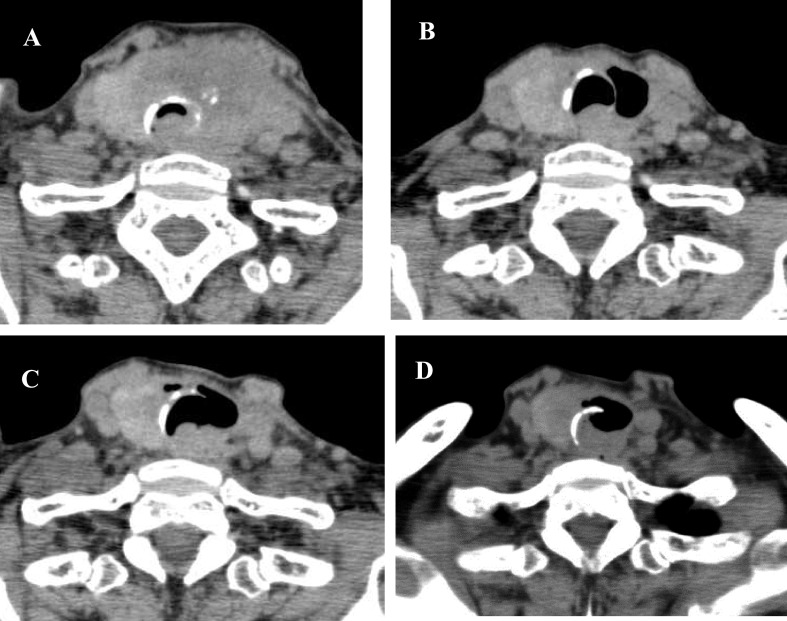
CT scans of patient’s neck. A: First day of hospitalization. B:
